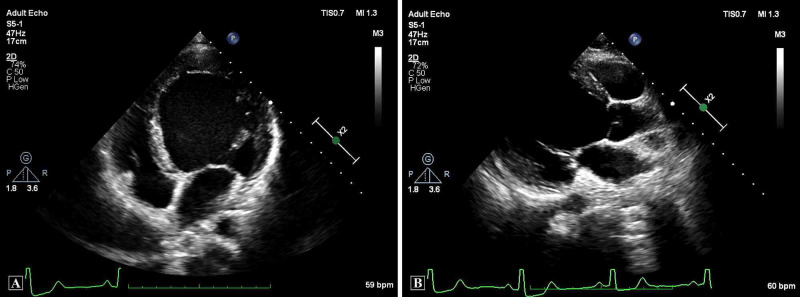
Transthoracic echocardiography at presentation. Apical four-chamber view (A) and parasternal long-axis view (B) showing dilated LV. The internal dimensions of the LV were 69 mm in diastole and 62 mm in systole. LV, left ventricular
